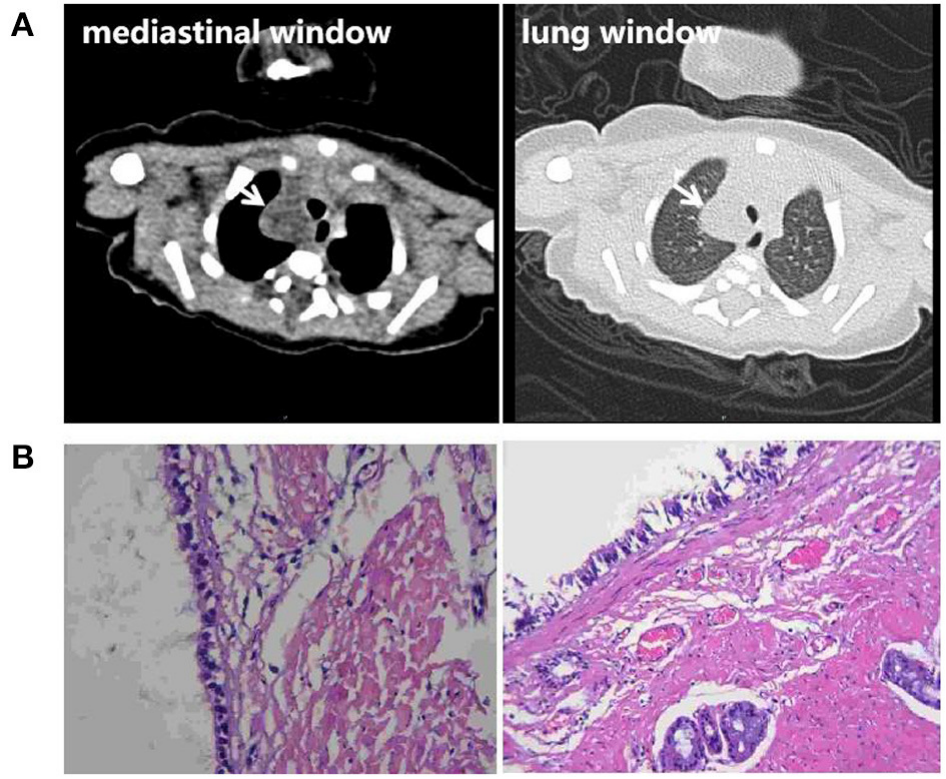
(A) Chest CT scan of the newborn 3 days after birth. An irregular low-density lesion with a diameter of about 16 mm was seen in the upper right mediastinum, with a slightly unclear boundary. The CT value was about 8 Hu. No abnormal thickening of the pleura was observed, and no signs of effusion were observed in the pleural cavity. (B) Postoperative pathological findings show a bronchogenic cyst.
